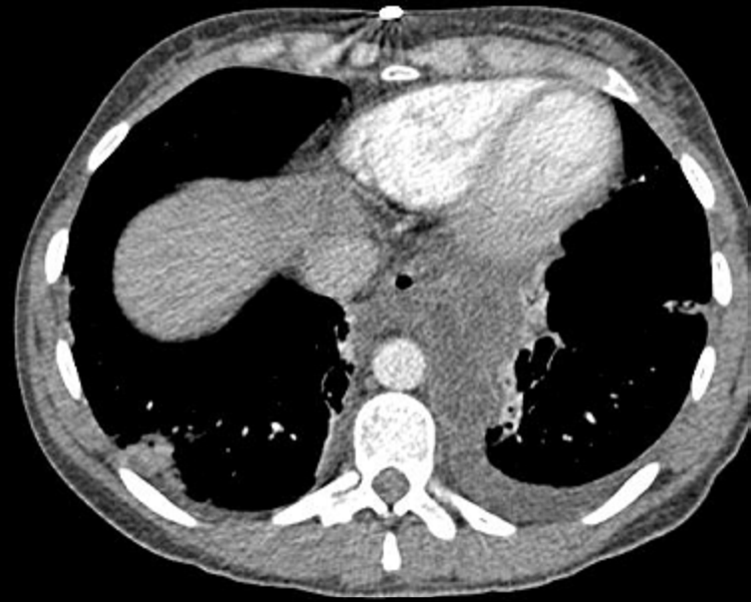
CT-scan showing pancreatic pseudocyst and its mediastinal extension.
